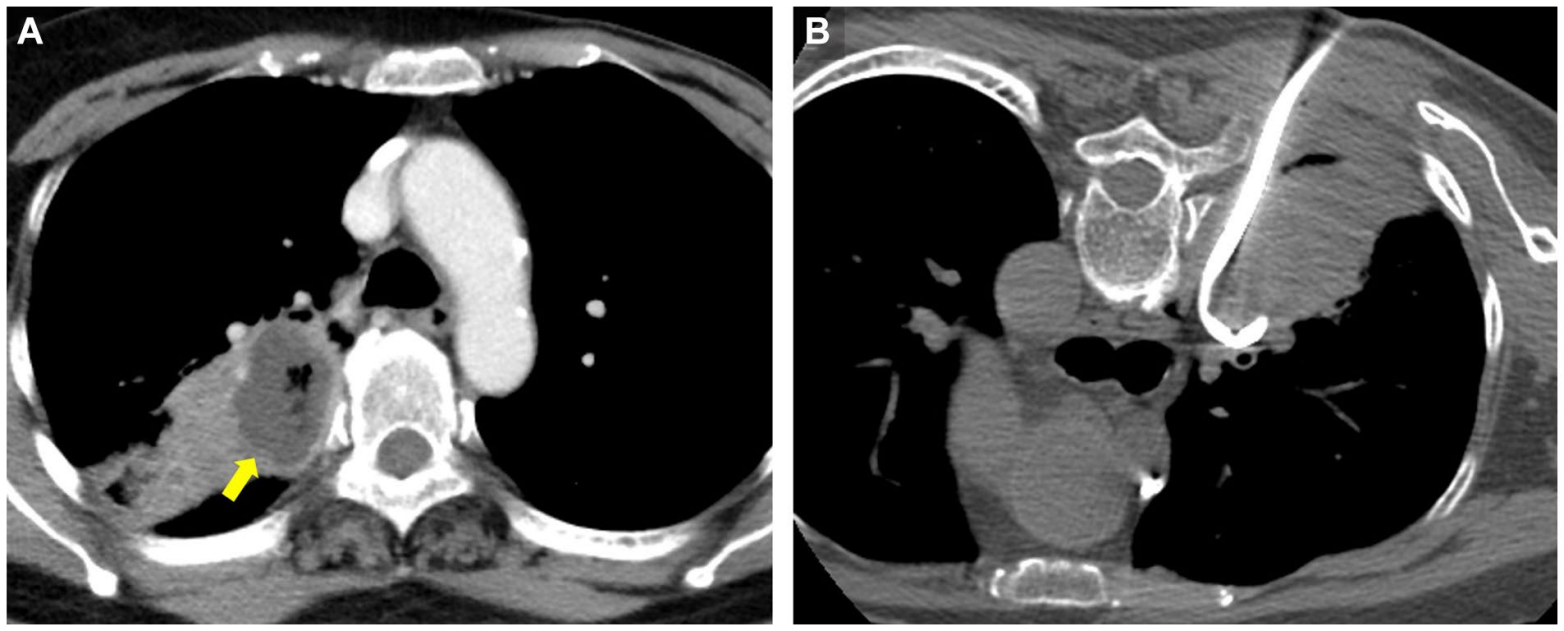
Representative contrast-enhanced CT (CECT) images with liquefied lung abscess in a 69 years-old woman who presented with fever and cough for 2 weeks. (A) CECT shows a 3.7 cm low-attenuation necrotic region (arrow) with gas formation. (B) CT-guided PCD was performed after CECT at the same day.
